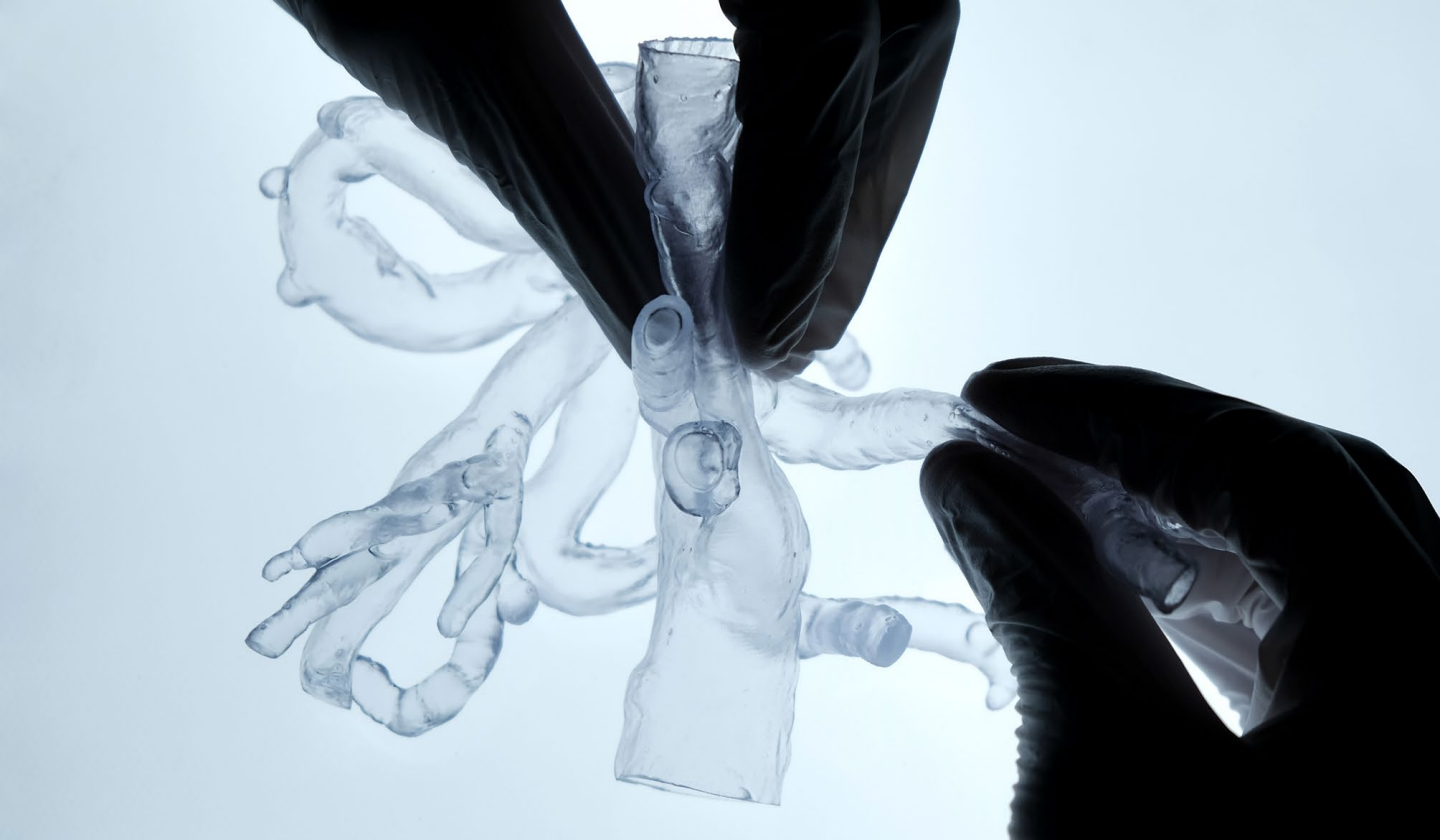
Proof of flexibility and transparency of a CT-derived vascular model, printed with flexible resin. The aorta and its branches are easily compressed with two fingers, demonstrating the flexible quality of this novel 3D-printing material. Transparency is high and can be further improved as demonstrated by background lighting with a LED panel
